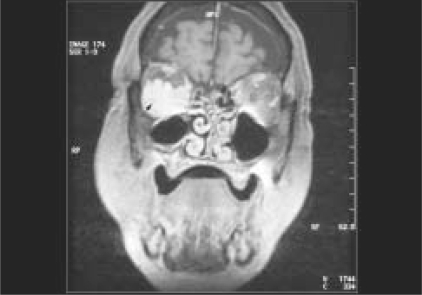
T2-weighted MRI scan reveals an intensely enhancing irregular mass lesion in the inferior orbit within which several flow voids can be observed (arrow)
